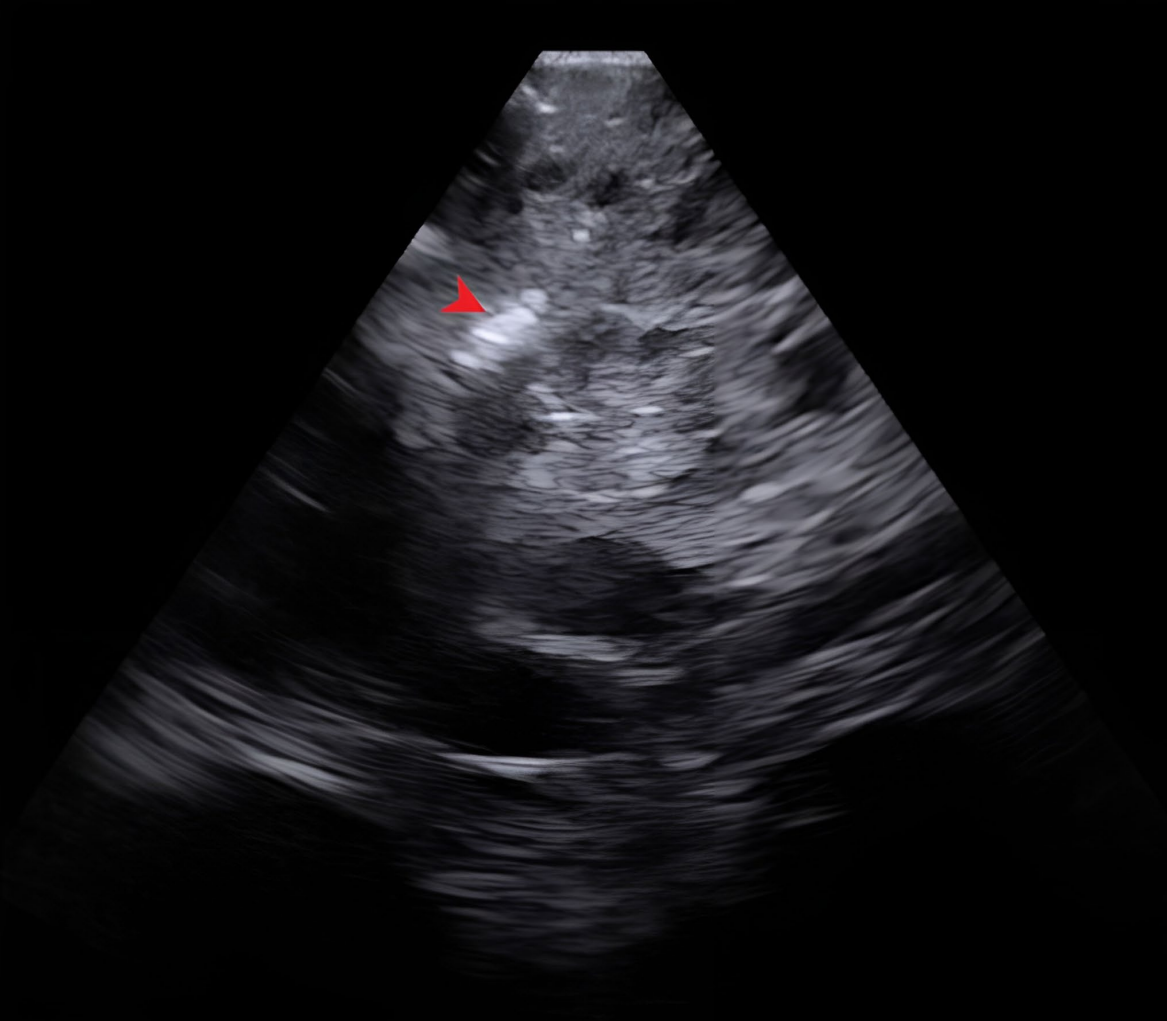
Intraoperative ultrasound images of Case 2 (before incising the dura) showing a heterogeneous lesion with few very hyperechoic areas suggestive of calcific foci (red arrowhead) along with the acoustic shadow. Hypoechoic cystic areas are also seen
